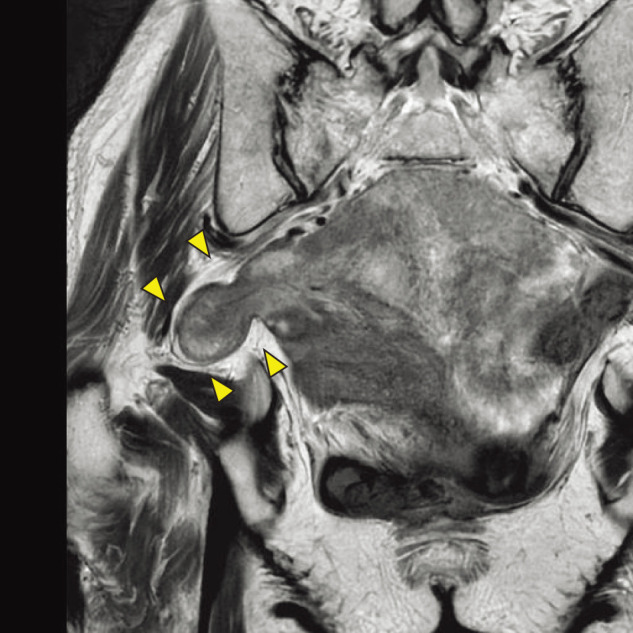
MRI findings. MRI showed a hernia orifice cranial to the sacrospinous ligament, consistent with a greater sciatic foramen hernia (arrowheads).
